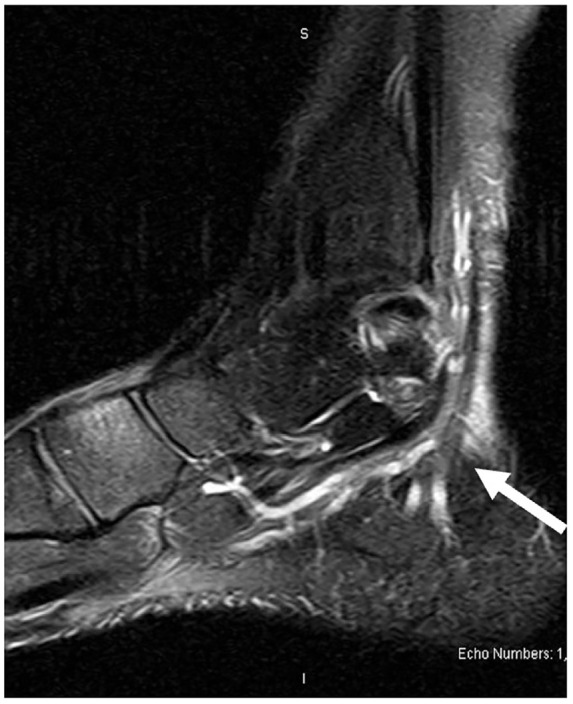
Magnetic resonance imaging: sagittal view.
